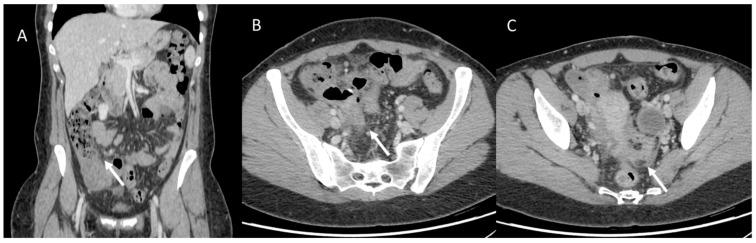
Woman with operated appendiceal tumor ((A): in coronal plane, arrow). CT assessment of peritoneal carcinomatosis ((B,C), arrow).
